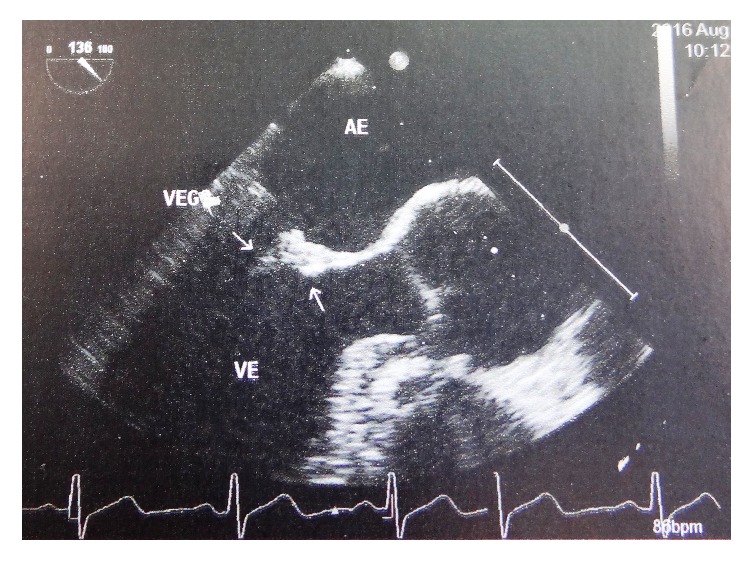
Vegetation on the anterior leaflet of the mitral valve shown on transesophageal echocardiogram.
